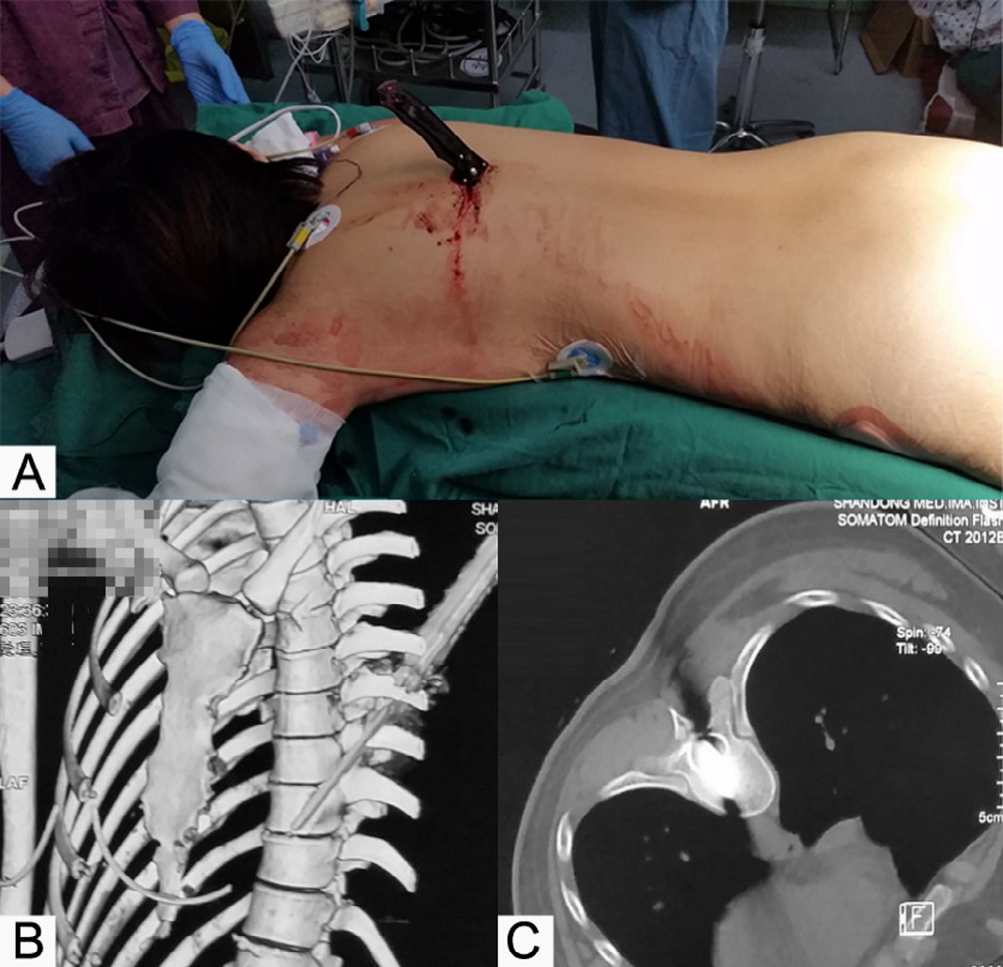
Case 1 (25‐year‐old female). (A) the patient was assaulted with a sharp knife; (B) three‐dimensional CT reconstruction and (C) coronal CT revealed a foreign body extending through the spinal canal and terminating in the thoracic cavity
